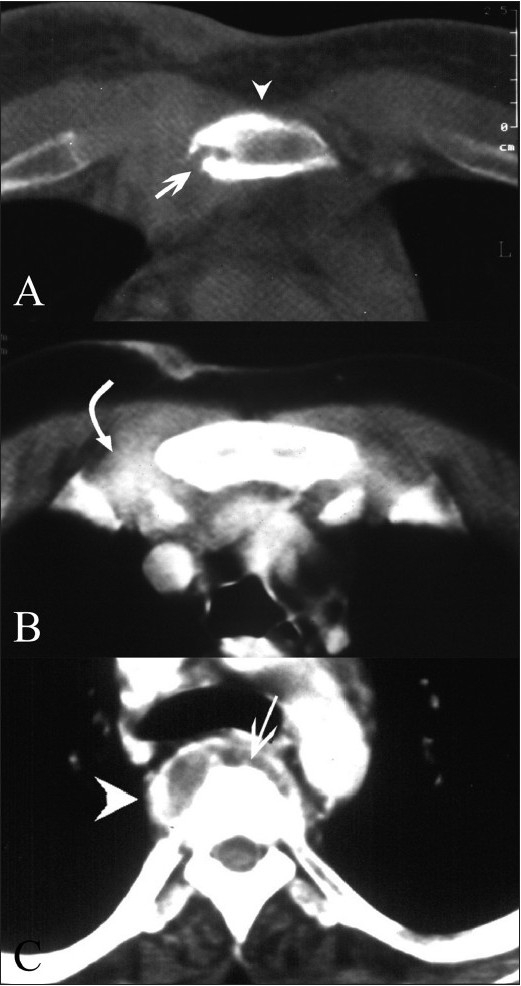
Sternal tuberculosis – patient 1. Contrast-enhanced CT scans show erosion (arrow) and sclerosis (arrowhead) of the sternum. The adjoining right parasternal soft tissue shows thickening with dense enhancement (curved arrow). Vertebral destruction (arrow in C) and a paravertebral abscess (arrowhead in C) are also noted
